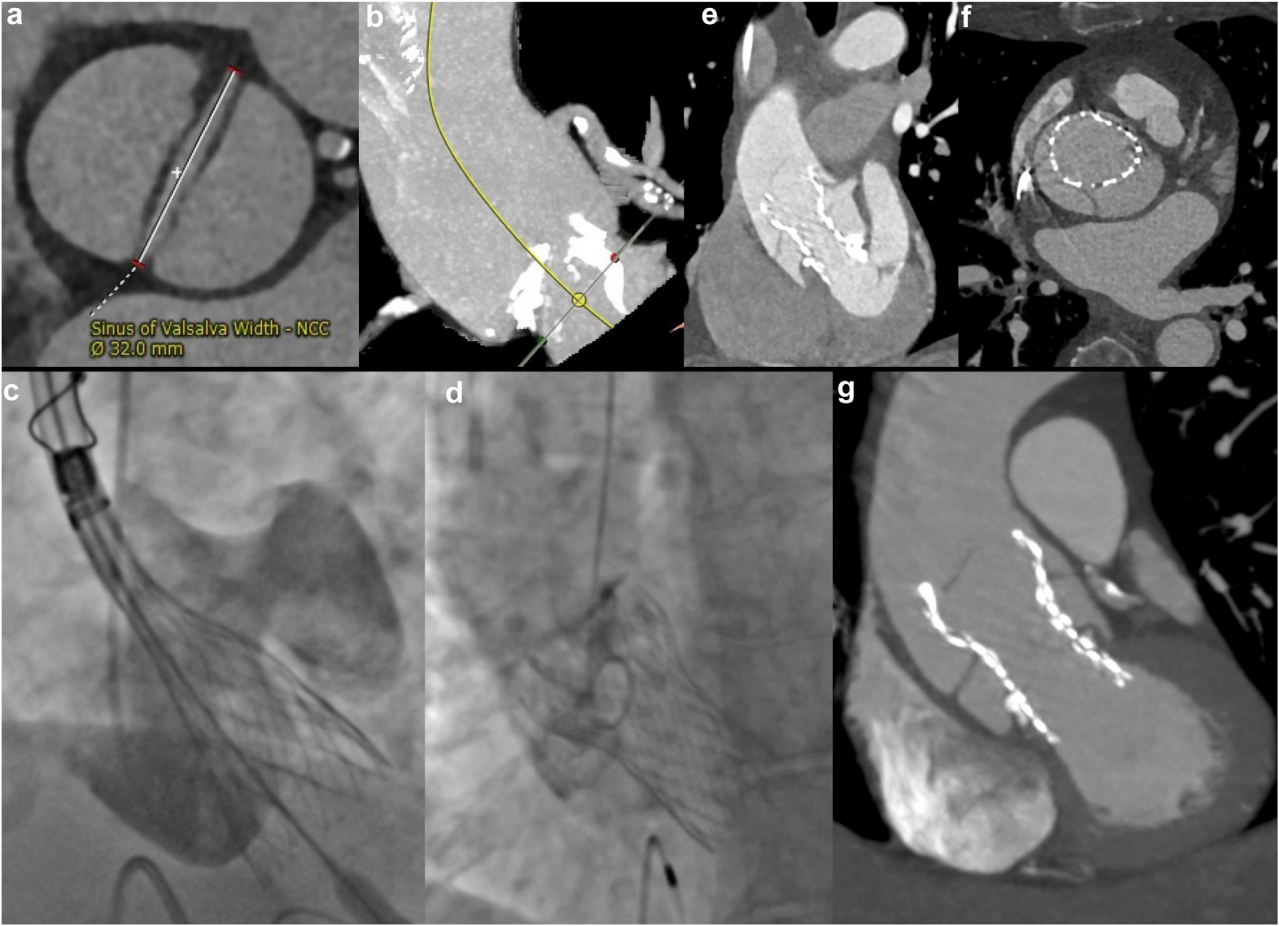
Iatrogenic aortic root dissection. (a) Cardiac CT: bicuspid aortic valve and (b) Heavy calcifications within aortic annulus and LVOT; (c) Aortogram: sinotubular aortic dissection during TAVR deployment; (d) Postdeployment aortogram: dissection pinned by TAVR cage; (e and f) Postprocedure CTA: sinotubular dissection; (g) 6-week follow-up CTA: stable dissection flap without extension. Abbreviations: CT, computed tomography; LVOT, left ventricular outflow tract; TAVR, transcatheter aortic valve replacement.
